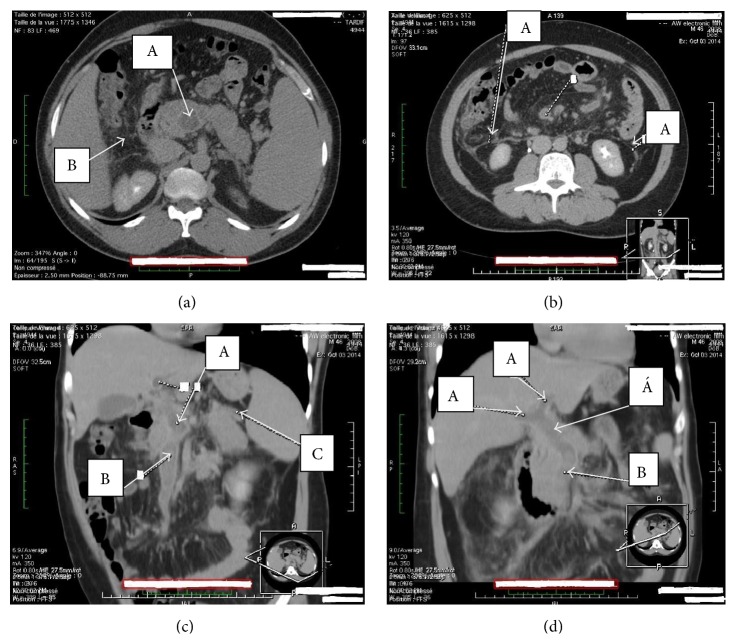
Abdominal contrast-enhanced CT scanner (hospital admission): (a) an edematous pancreas in (A) the superior mesenteric vein thrombosis and (B) the infiltration of the peripancreatic fat. (b) (A) Inflammatory flows. (c) (A) The portal venous thrombosis, (B) the superior mesenteric venous thrombosis, and (C) the splenic venous thrombosis. (d) (A) The thrombosis of the left and right branches of the portal vein and (B) the superior mesenteric venous thrombosis and distal branches. Light Speed CT-16 (GE Medical Systems, USA). Contrast: iobitridol 300 mg/mL (Xenetix, Guerbet, Roissy CDG Cedex, France). With the permission of the Cathedral Medical Center (CMC), Yaoundé.
